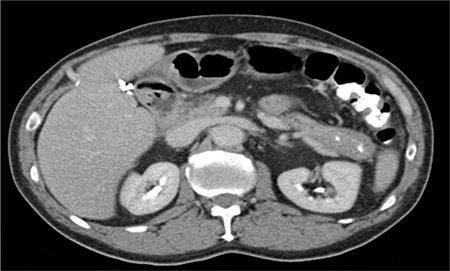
Computed tomography scan of the pancreas. The image demonstrates a hypoattenuating pancreatic mass.
